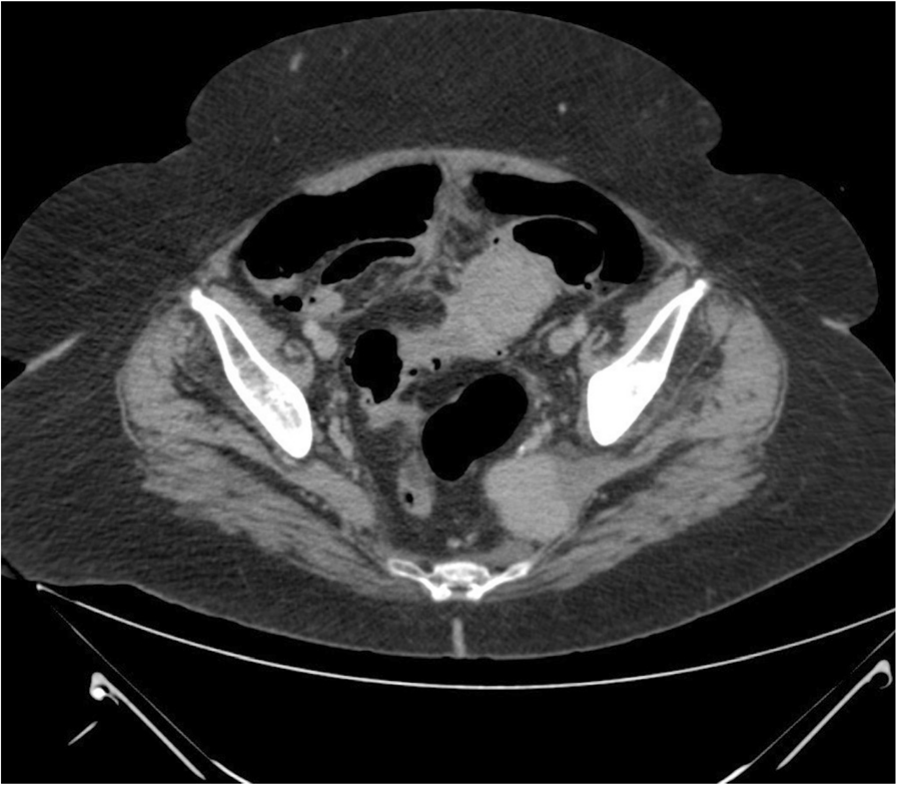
Axial computed tomography after administration of intravenous contrast shows enhancement of the lesions
